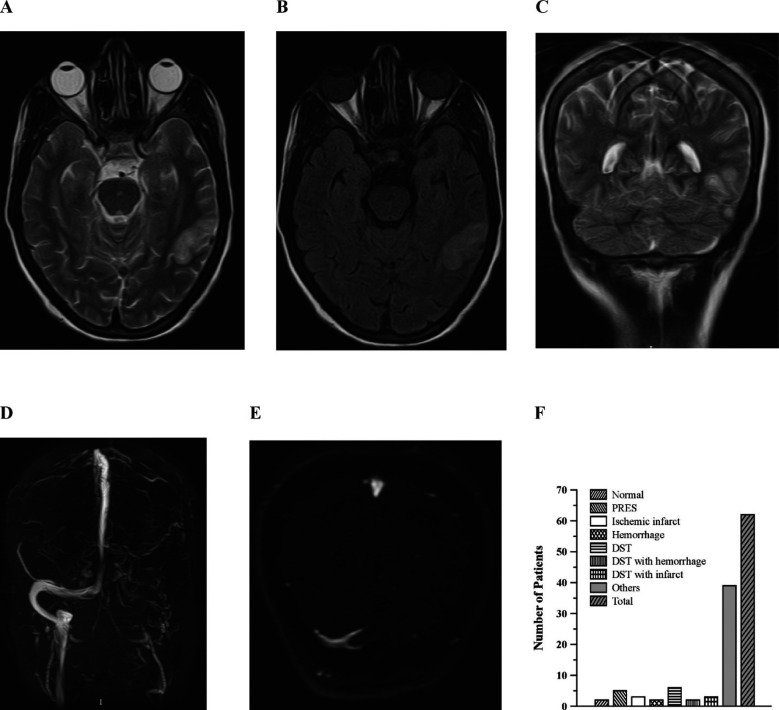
Findings of MRI Brain. A; T2W axial image, B; FLAIR axial image both showing hyper intense area in left temporal lobe, C; T2W coronal image showing left temporal lobe hyper intensity and loss of signal void in left transverse sinus, D; 3D VR MRV and E; Axial MRV reveals non visualization of left transverse & sigmoid sinus as well as internal jugular vein with evidence of multiple collateral vessels confirming left transverse and sigmoid sinus thrombosis with temporal lobe infarct, F; graphical presentation of various MRI findings.
